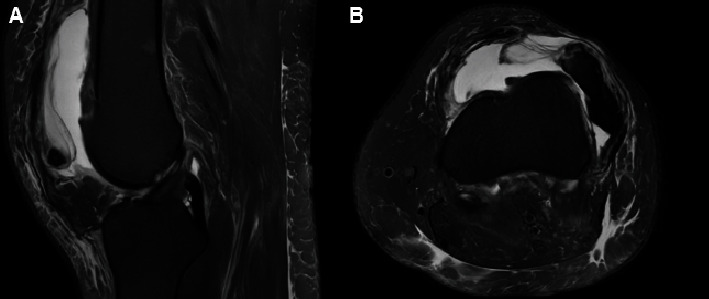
Preoperative MRI. T2 MRIs of the patients left knee. (A) Sagittal T2 MRI demonstrating a large joint effusion with a large fracture fragment located anterior to the femoral trochlea. (B) Axial T2 MRI demonstrating a large joint effusion with dysplastic femoral trochlea and a complete lateral dislocation of the patella.
